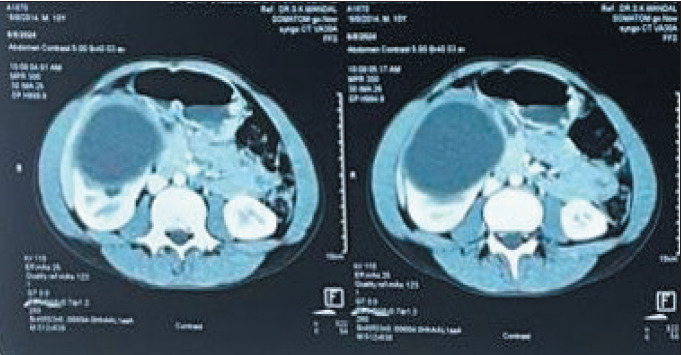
CT abdomen showing a cystic lesion in lower pole of the right kidney.
